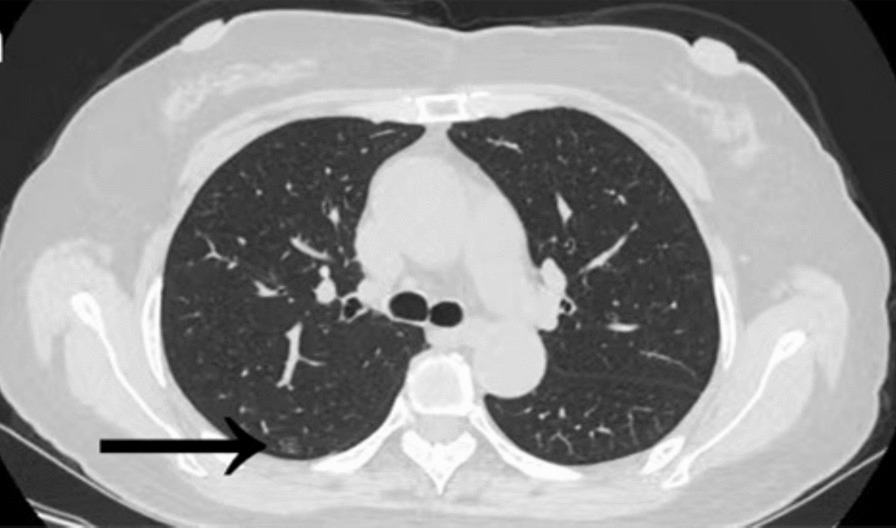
Chest CT scan showing a 4–5 mm GGN in the right upper lobe (arrowheads)
